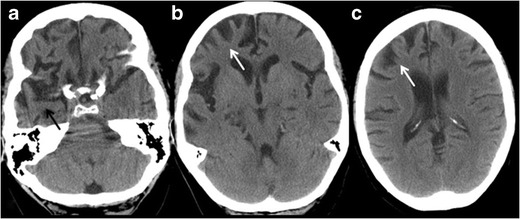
Behavioural variant Frontotemporal dementia (bvFTD). A 53-year-old male with progressive personality change and behavioural problems with cognitive decline. a, b & c axial CT sections of brain show severe right frontal lobe atrophy (*white arrow*), as well as some atrophy of right anterior temporal lobe (*black arrow*)
